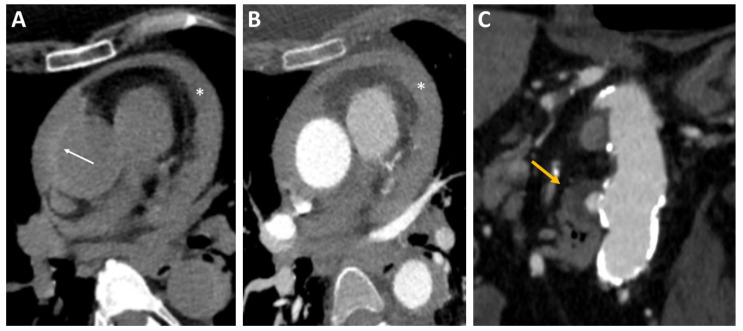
Intramural hematoma on CT using (A) non-contrast CT and (B) CT angiography: crescentic, high-attenuating regions of eccentrically thickened aortic wall on non-contrast CT (arrow). A diffuse pericardial effusion (*) was also visible in both scans. (C) Penetrating ulcer on CT: CT angiography image showing a penetrating ulcer of the descending aorta as a contrast-filled, out-pouching into the thickened aortic wall (arrow). CT, computed tomography.
